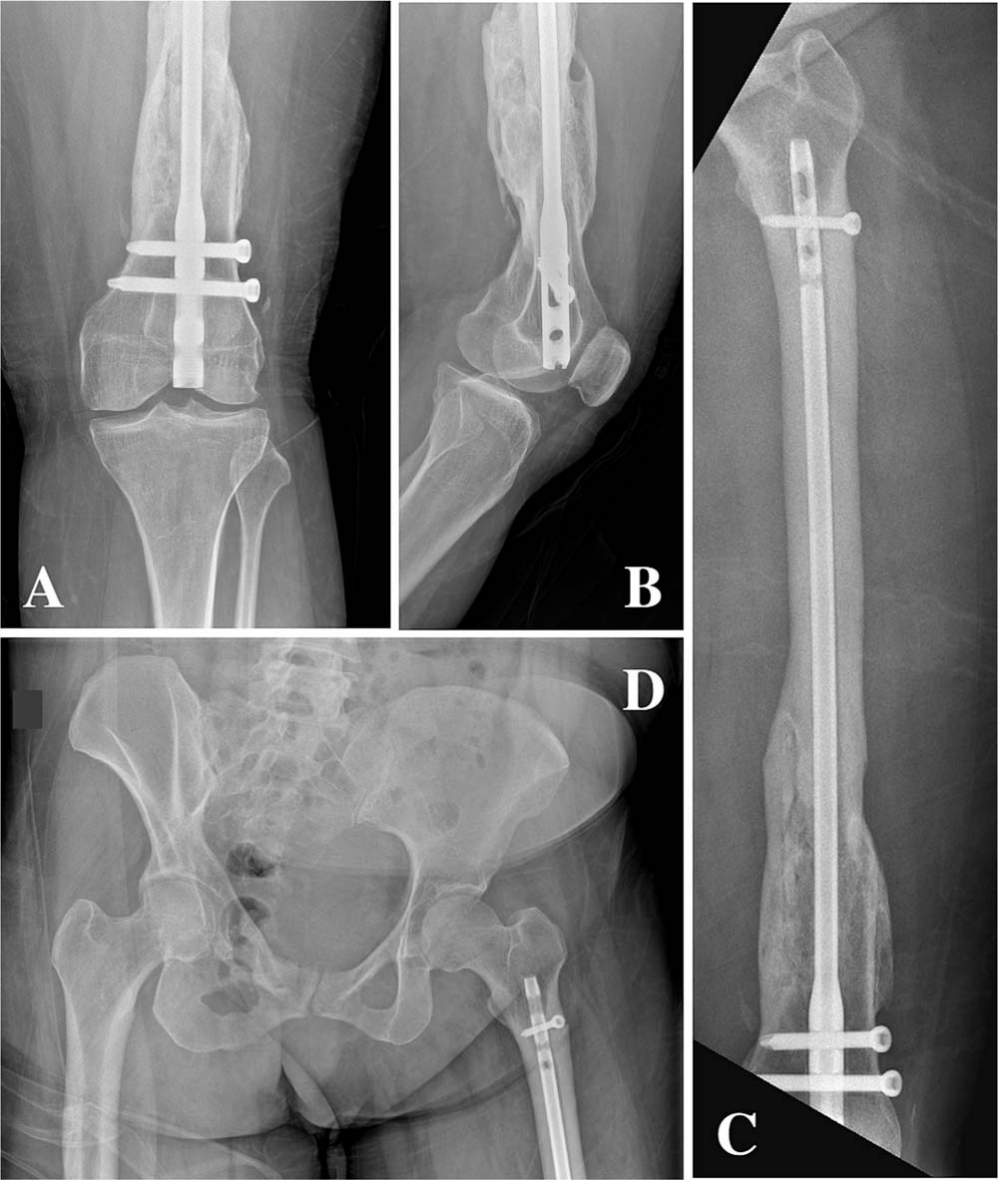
(A, B) Anteroposterior and lateral radiographs showing hypertrophic non-union of the distal femur with retained intramedullary nail and locking screws. (C) Anteroposterior view demonstrating a femoral nail inserted via a distal entry point with abnormal proximal extension. (D) Anteroposterior pelvis radiograph showing the antegrade-design nail extending proximally from a retrograde entry point, terminating proximal to the lesser trochanter.
